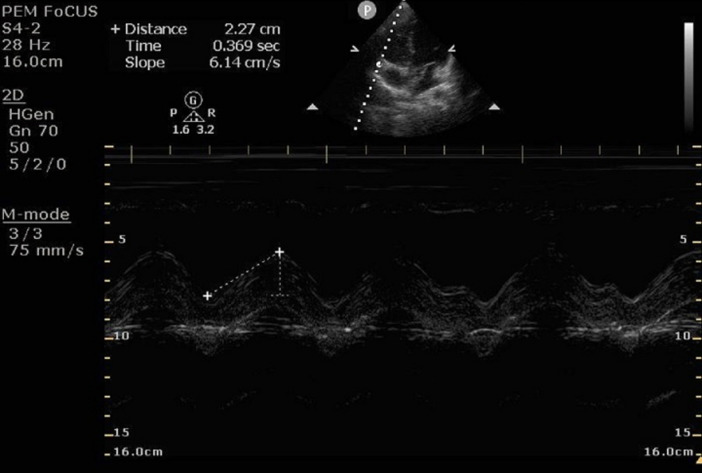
TAPSE measurement obtain in the apical four chamber view. Movement of the lateral tricuspid annulus during the cardiac cycle is measured. The peak to trough measurement is 2.3 cm
